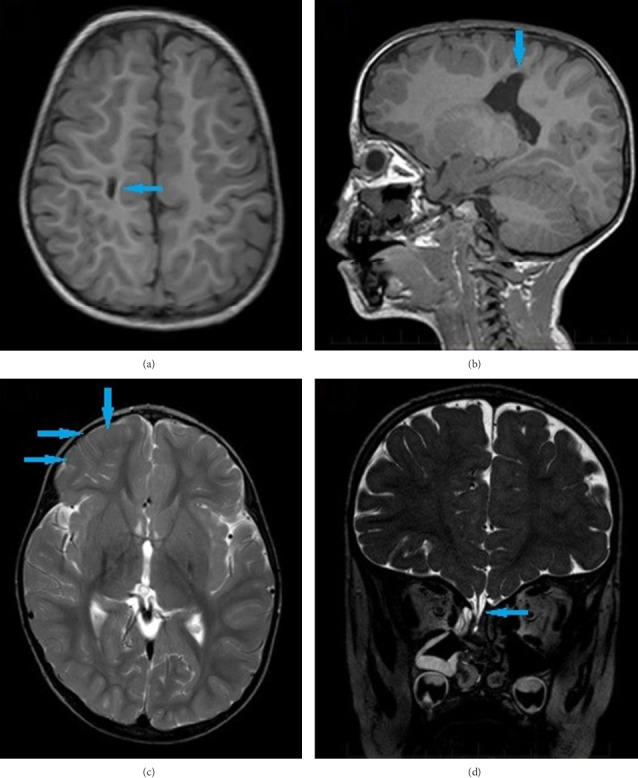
T1-weighted images (a and b) evidence motor pathway injury (arrows). T2-weighted image in (c) shows sequelae of prefrontal cortical and subcortical injury (arrows). 3D DRIVE T2-weighted image in (d) highlights the herniation of the rectus gyrus of the right frontal lobe through the base skull defect (arrow).
